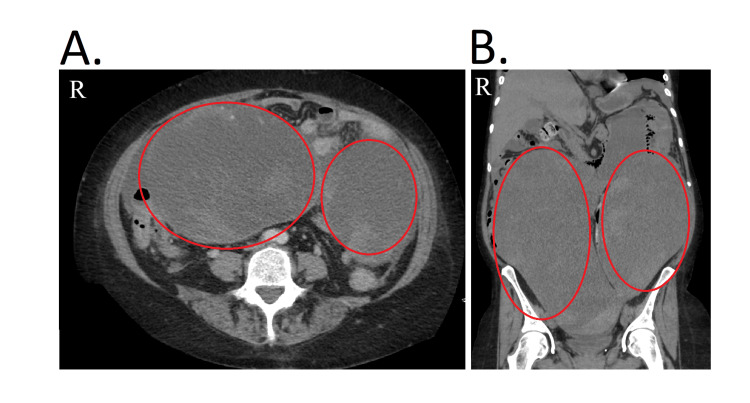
Computed tomography (CT) of the abdomen and pelvis without contrast. (a) CT transverse view shows approximately right lower abdominal well-defined heterogeneous enhancing soft-tissue mass measuring 12.7 × 16.5 × 20.9 cm and left lower abdominal well-defined heterogeneous enhancing soft tissue mass measuring 13.3 × 8.6 × 13.2 cm noted by the red circles. (b) CT coronal view of Figure 2a.
